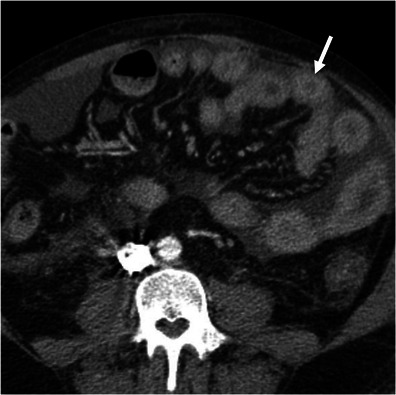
Shock bowel in a patient with significant haemorrhage due to bleeding oesophageal varices. Axial contrast-enhanced CT scan shows thickened hyperattenuating small bowel loops (*arrow*) due to the increased vascular permeability in the context of severe hypovolaemia. Also note engorgement of the mesenteric vessels and small volume ascites
